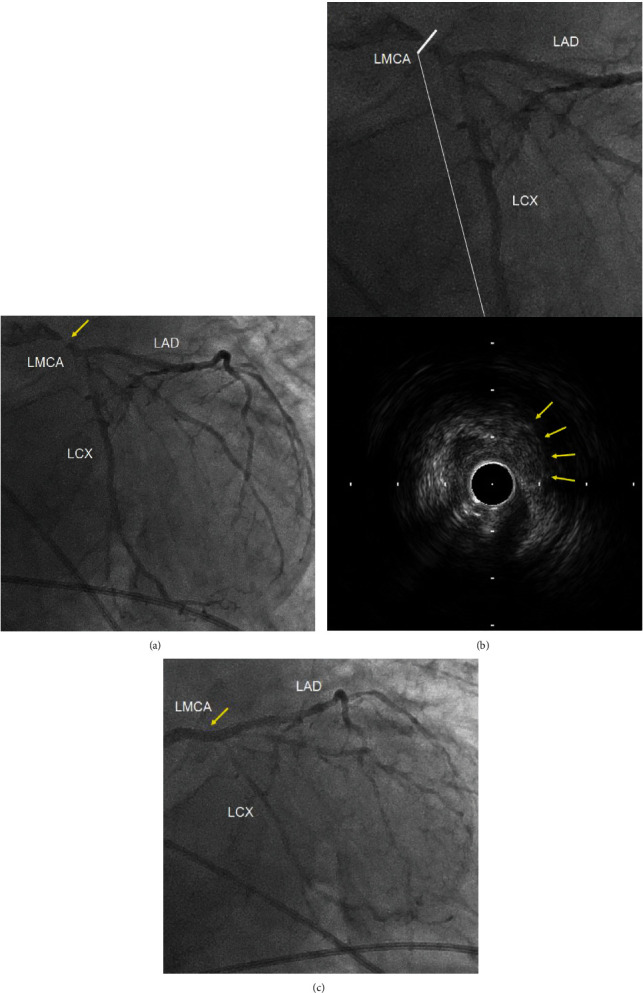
(a) Emergent coronary angiography. Right anterior oblique caudal view showing a severe stenosis of mid LMCA (arrow). LMCA: left main coronary artery; LAD: left anterior descending artery; LCX: left circumflex artery. (b) Intravascular ultrasound study. The section at the white line showing low attenuation plaque around the entire circumference of the vessel with ulceration (arrows) in the mid LMCA. LMCA: left main coronary artery; LAD: left anterior descending artery; LCX: left circumflex artery. (c) Final coronary angiography. Right anterior oblique caudal view showing no significant LMCA stenosis with grade 3 TIMI flow after stent implantation (arrow). LMCA: left main coronary artery; LAD: left anterior descending artery; LCX: left circumflex artery.
